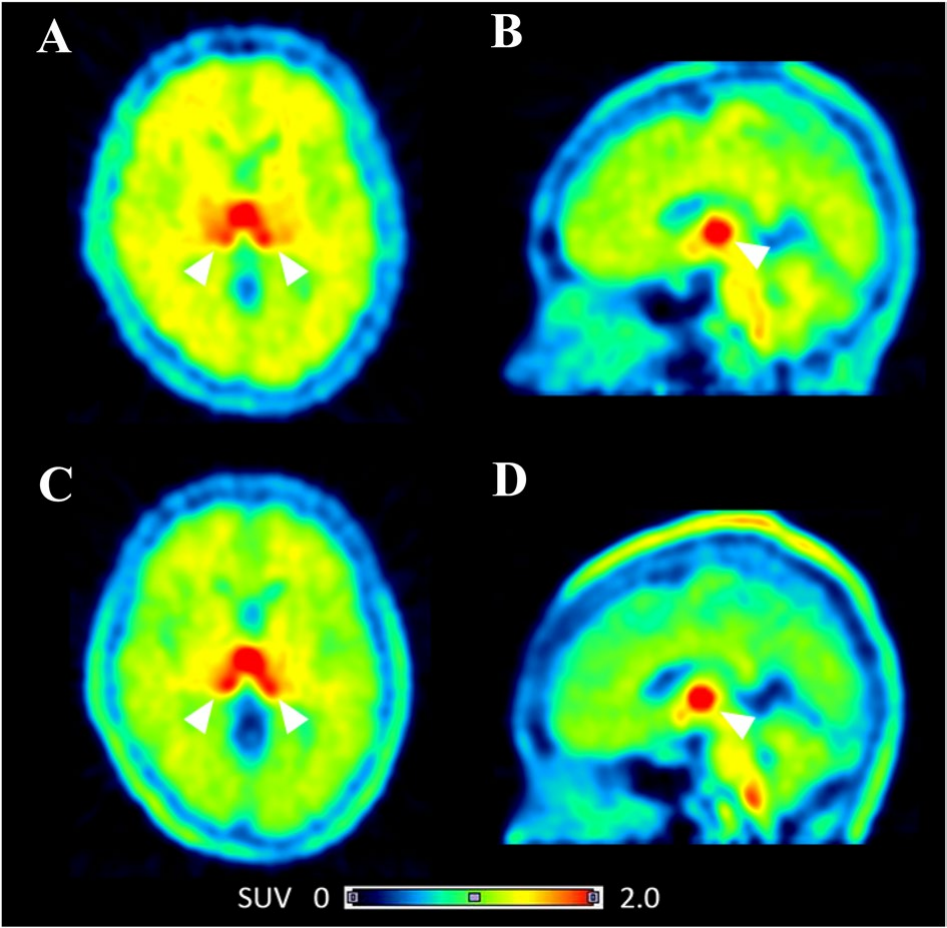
The representative SUV images of [^11^C]cetrozole (A,B) and [^11^C]iso-cetrozole (C,D) in the brains of similar individuals (A,C transaxial slices; B,D sagittal slices). Arrow heads indicate the thalamus.
